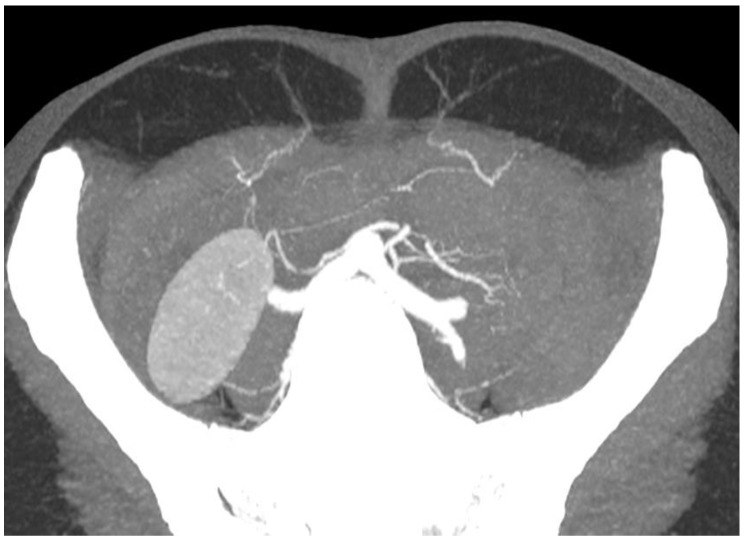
Axial CTA slice of the abdomen with maximum-intensity projection applied displaying the vasculature of the anterior abdominal wall and the intramuscular course of the medial row perforators.
